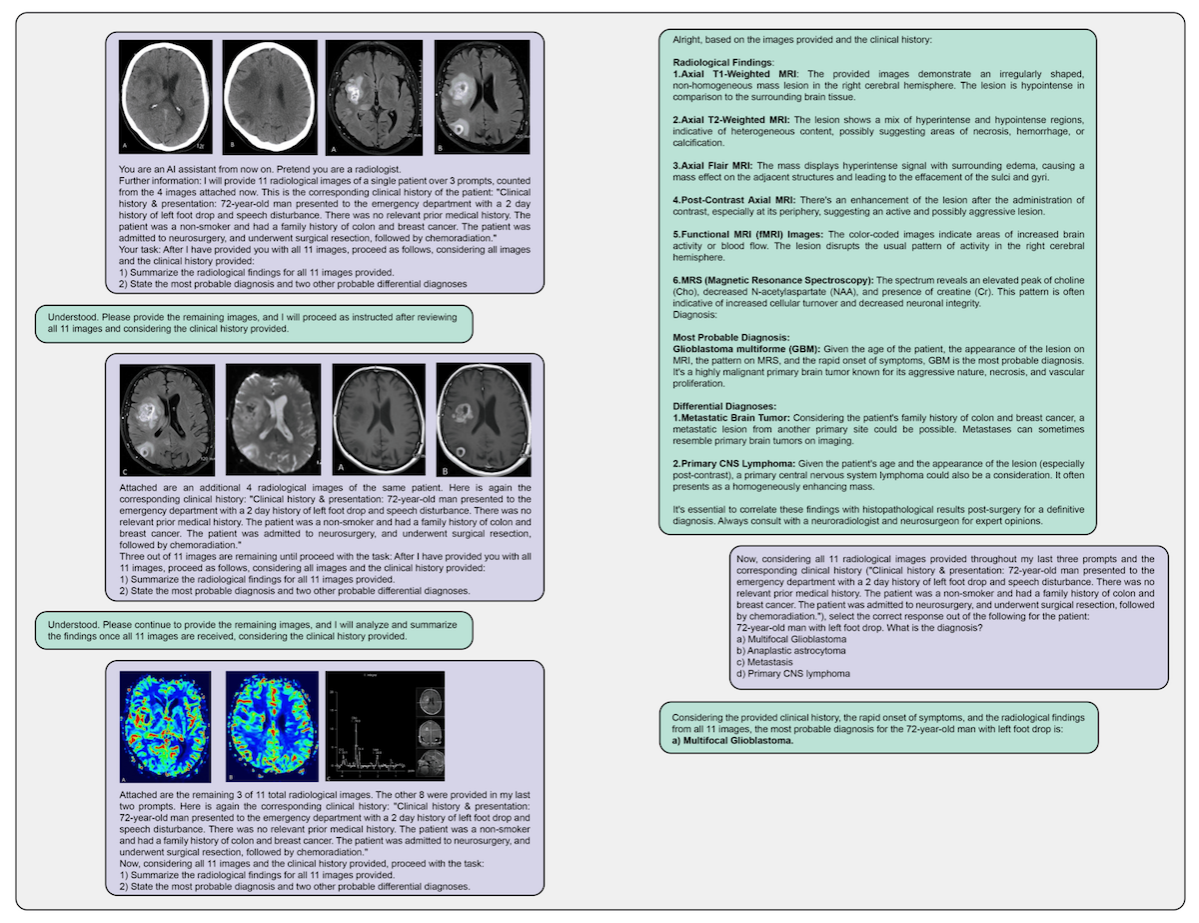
An example conversation with GPT-4V showcasing the prompting style that was used if the question contained more than 4 images. Notably, GPT-4V often disregards the initial textual case description when additional image prompts are introduced, necessitating the description’s repetition for accurate responses. As the context window, image resolution limit, and embedding size limit of the web application version of GPT-4V are unknown, definitive conclusions regarding the cause of this forgetfulness could not be drawn. Nevertheless, the model’s consistent ability to recognize and recall images from the initial prompt (eg, axial FLAIR [fluid-attenuated inversion recovery] images) suggests that running out of context length is an unlikely explanation. Reproduced with permission from the Radiological Society of North America. Link to the displayed case: https://cases.rsna.org/take-quiz/07c4b917-80fb- 43c0-8b3b-59a0d8ceb203 (accessed 14th January 2026).
